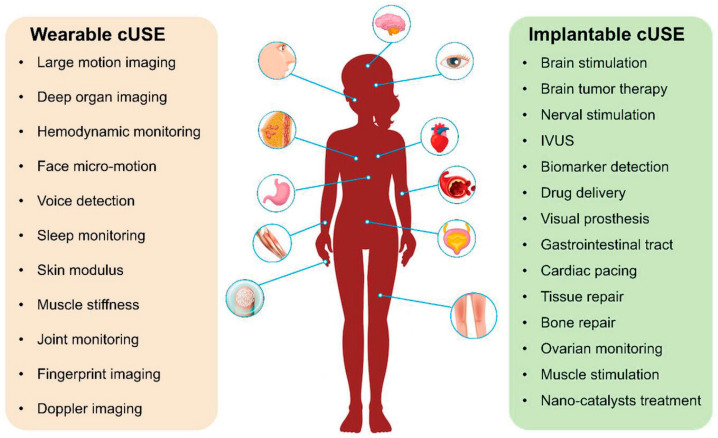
Wearable and implantable conformable ultrasound electronics (cUSE) for various healthcare uses. Reproduced with permission from ref. [214]. Copyright 2023 Wiley-VC Open Access.
